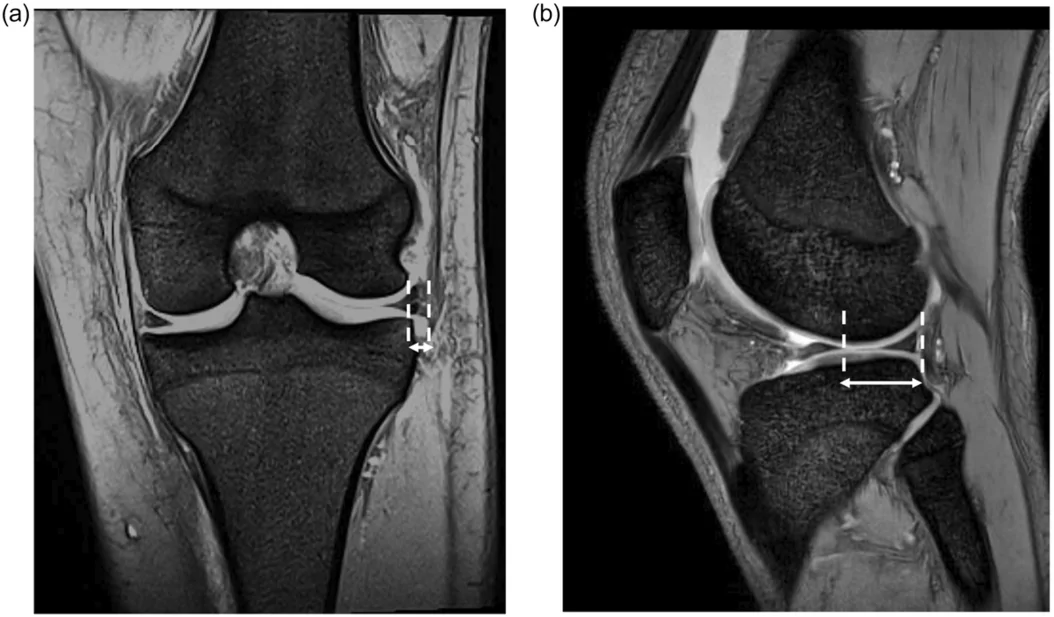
Measurement of lateral meniscal extrusion on MRI. (a) Coronal image showing measurement of coronal lateral meniscal extrusion. (b) Sagittal image showing measurement of sagittal lateral meniscal extrusion. MRI, magnetic resonance imaging.
